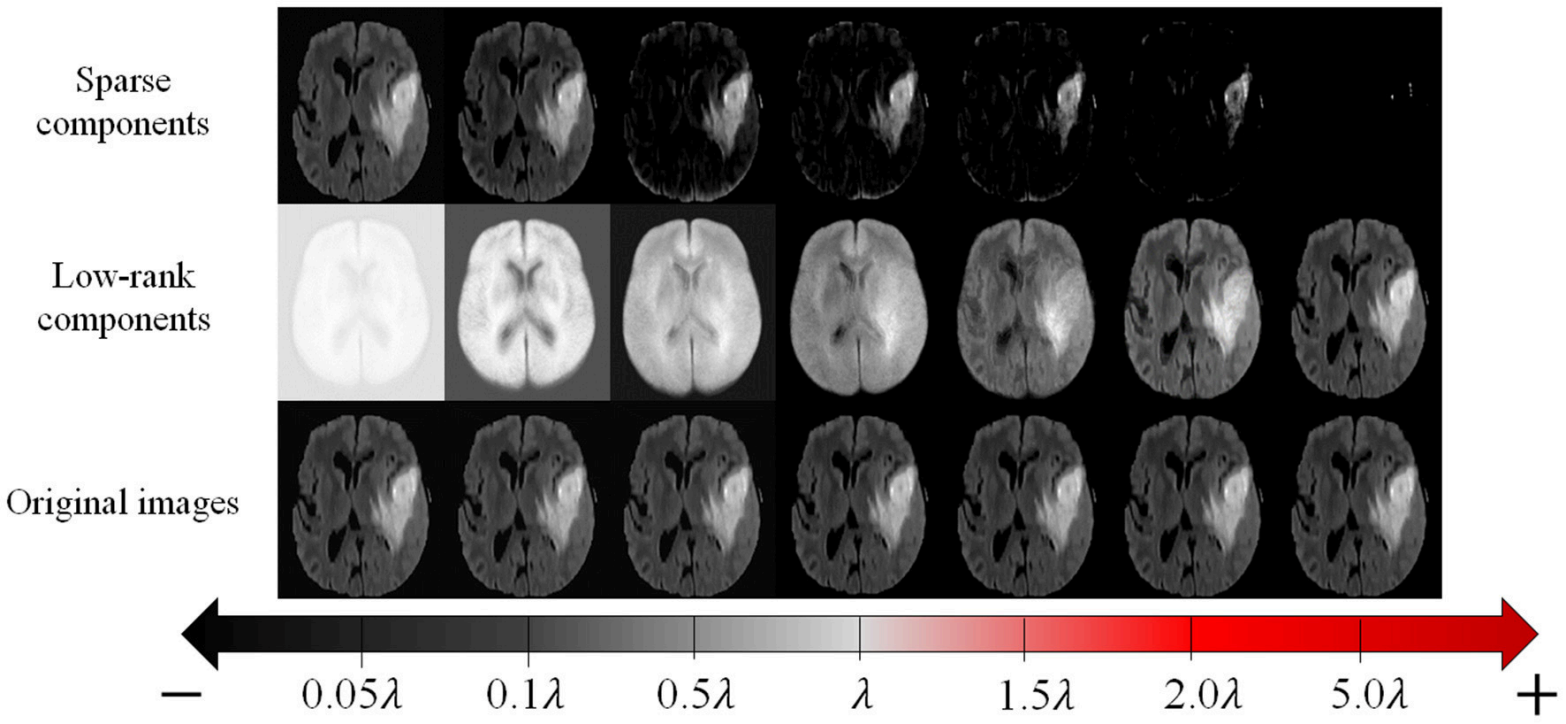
The original MR images (bottom row), and corresponding recovered low-rank components (middle row) and sparse components (top row) given by conventional LSD method, with different values of regularizing parameter λ.
